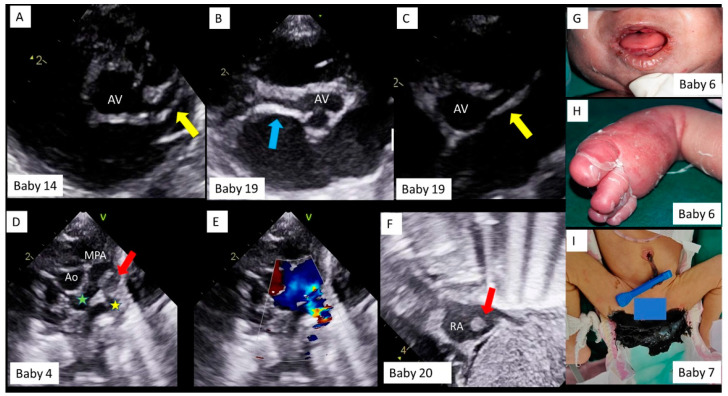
Echocardiography and clinical findings in neonates with MIS-C. Baby number sequence is the same as in Table 3. Transthoracic echocardiography, parasternal short axis view in Baby #14 (A) and Baby #19 (B,C). The left main and left anterior descending coronary artery (yellow arrow) and the right coronary artery (blue arrow) are significantly dilated. AV = aortic valve. Transthoracic echocardiography and color doppler, parasternal short axis view in Baby #4 (D), showing aorta (Ao) and main pulmonary artery (MPA) bifurcation, with a large thrombus (red arrow) obstructing the left pulmonary artery (yellow star) origin and causing flow turbulence on color doppler (E), but normal flows across right pulmonary artery (green star). Transthoracic echocardiography subcostal bi-caval view in Baby # 20 (F), showing a thrombus (red arrow) in right atrium (RA). Baby #6, showing oral and muco-cutaneous lesions (G) and, pedal edema and skin peeling (H) and Baby #7 with black, tarry stools (melena, I).
